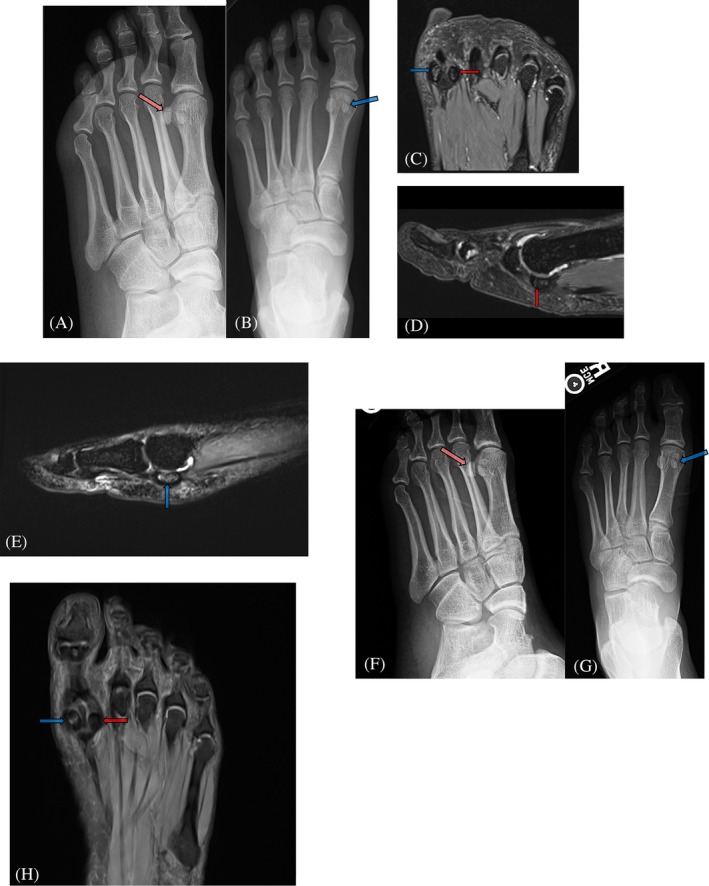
Left foot radiographs (A, B) and magnetic resonance images (C–E). Repeat 1‐year follow‐up left foot radiographs (F) and magnetic resonance images (G–H). (A) Linear lucency through the distal aspect of the fibular hallux sesamoid suspicious for nondisplaced fracture (red arrow). (B). Bipartite tibial hallux sesamoid (blue arrow). (C). Bone marrow edema involving the fibular hallux sesamoid with superimposed fracture line (red arrow). Bipartite tibial hallux sesamoid with associated bone marrow edema (blue arrow). (D). Fracture involving the fibular hallux sesamoid with scant surrounding bone marrow edema (red arrow). (E). Bipartite tibial hallux sesamoid with associated bone marrow edema, more pronounced at the distal pole (blue arrow). (F). Interval resolution of fracture lucency within the fibular hallux sesamoid (red arrow). (G). Bipartite tibial hallux sesamoid (blue arrow). (H). No fracture line or bone marrow edema identified within the fibular hallux sesamoid, consistent with healed stress fracture (red arrow). Interval resolution of bone marrow edema in the bipartite tibial hallux sesamoid (blue arrow).
